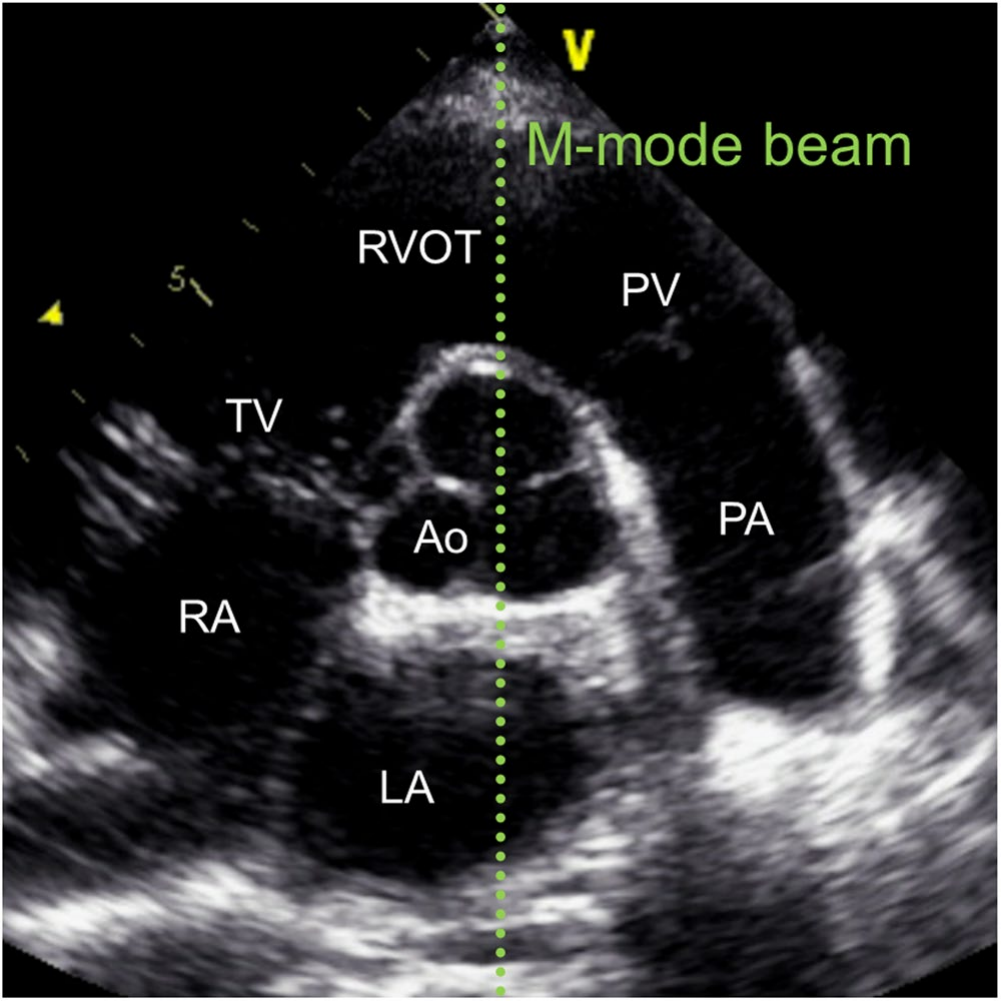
Parasternal echocardiographic B-mode image at the level of the valvular plane. The M-mode beam (light green) is directed through the center of the aortic root (Ao). LA, left atrium; PA, pulmonary artery; PV, pulmonary valve; RA, right atrium; RVOT, right ventricular outflow tract; TV, tricuspid valve
